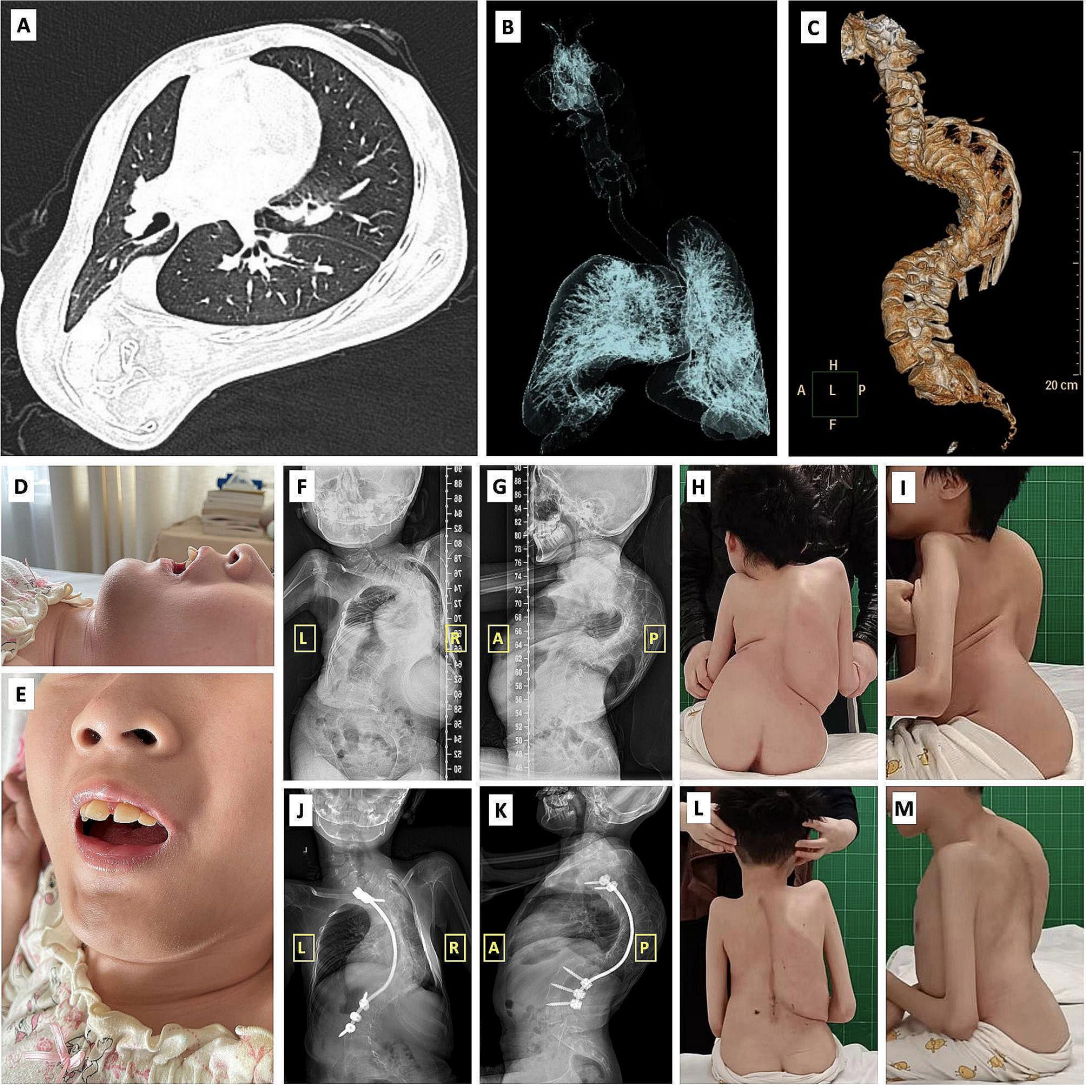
Clinical features of the patient. A, B) Chest CT and 3D airway tree reconstruction, showing thoracic asymmetry, bronchial stenosis, and underexpansion of the right lung. C) Preoperative 3D reconstruction of spine CT. D, E) Airway assessment. The girl is presented with very limited mouth opening for less than one finger. F, G) Preoperative X-ray images. H, I) Preoperative general observation shows severe kyphoscoliosis and multiple joint contractures. J, K) Postoperative X-ray images. L, M) Postoperative general observation shows improved kyphoscoliosis and sitting balance
